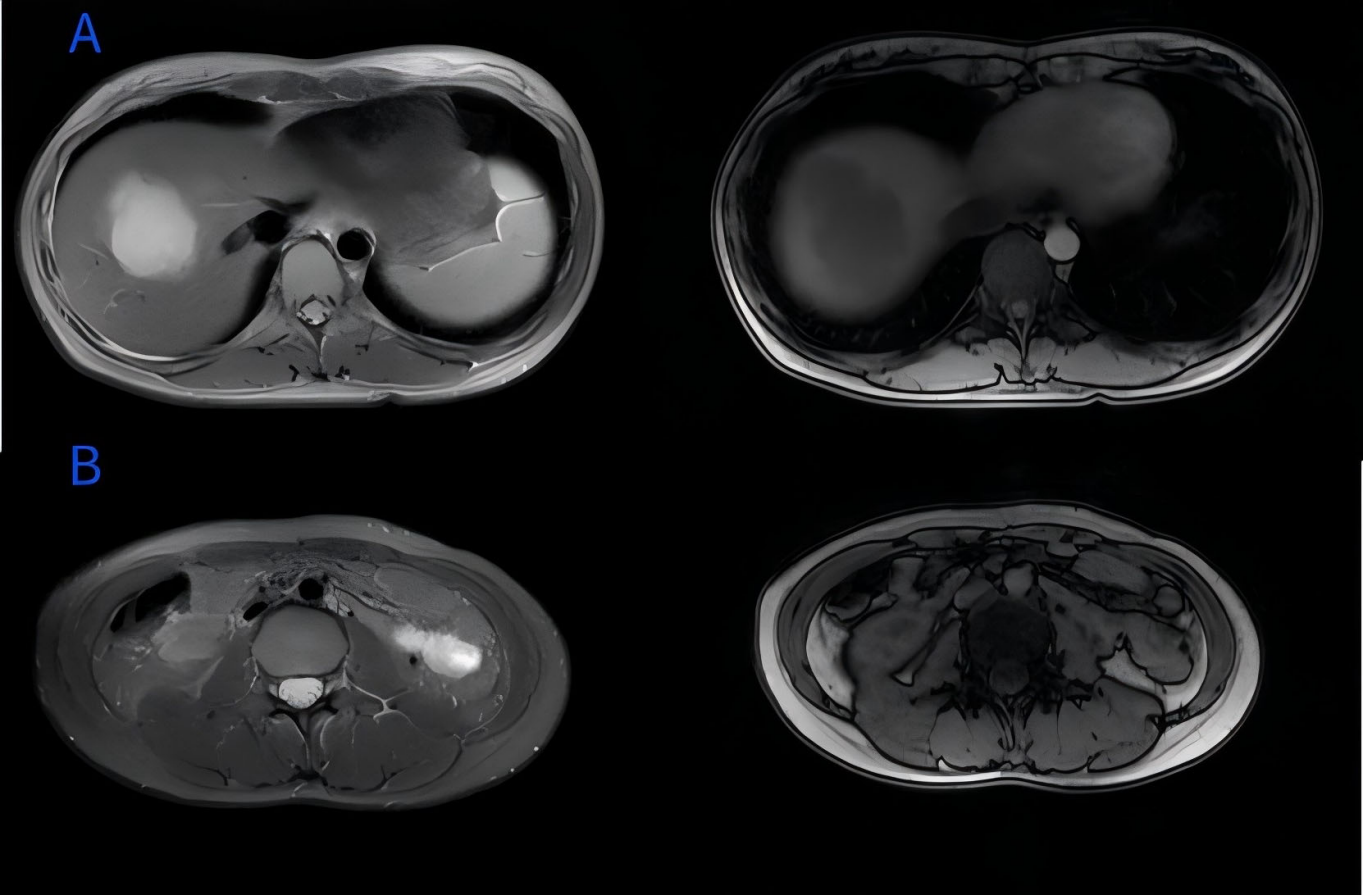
(A) Abdominal-enhanced magnetic resonance imaging (MRI) showed intrahepatic lesion with rim enhancement in S8 segment of liver; (B) abdominal MRI showed rectosigmoid mass with heterogeneous enhancement (red arrow).
